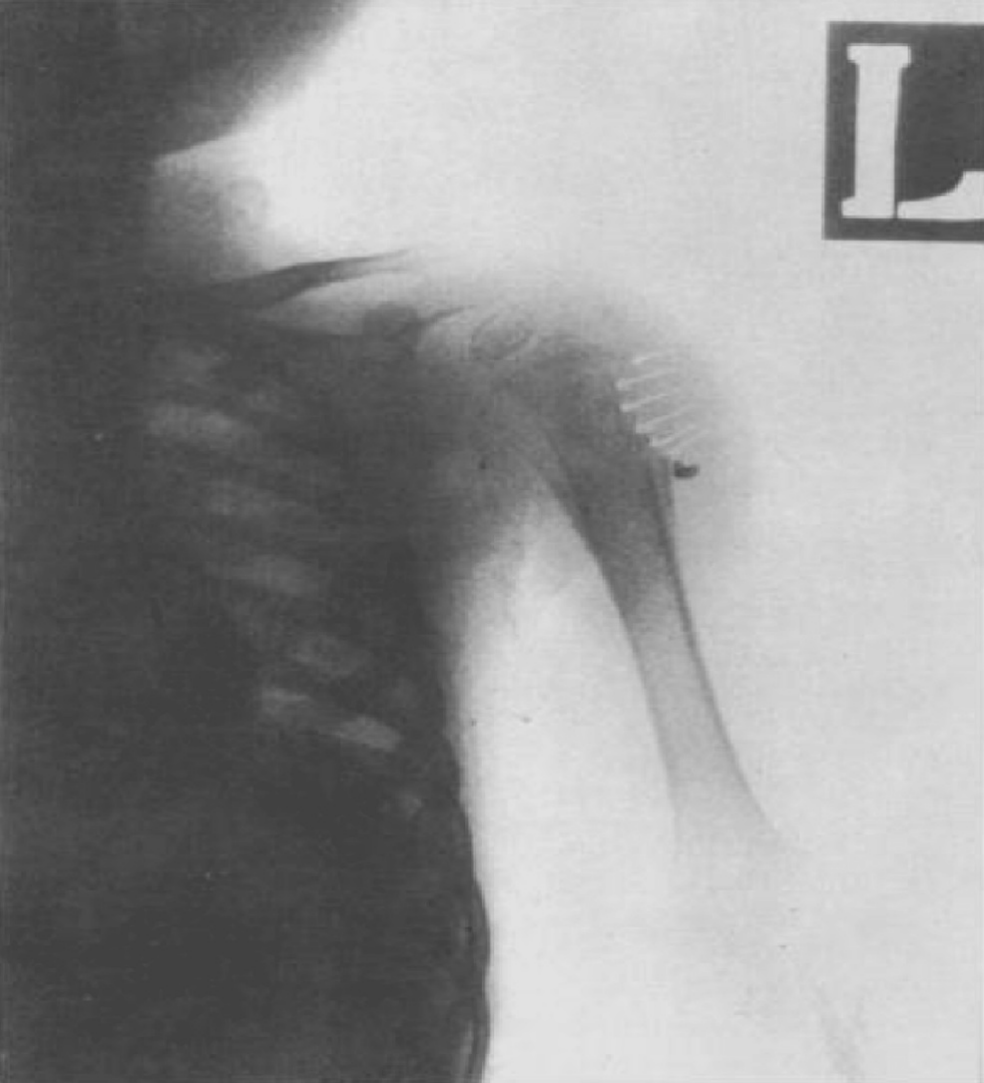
Plain radiograph showing destructive lesions in the left humeral metaphyseal area with an elevation of the periosteum and new bone formation
